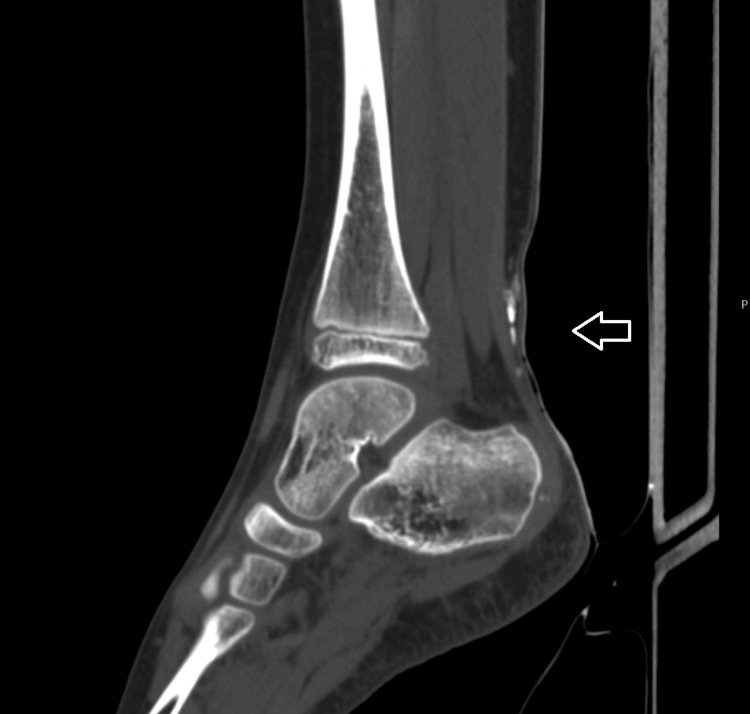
CT image on the sagittal plane showing a thin calcified lesion, located above the Achilles tendon, extending toward the sural nerve and vessel.
